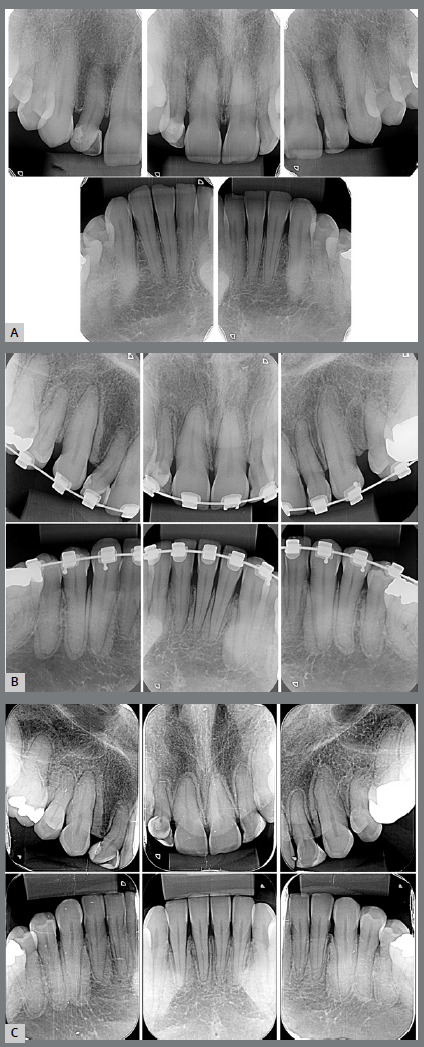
Beginning (A), two years after (B), and final periapical
